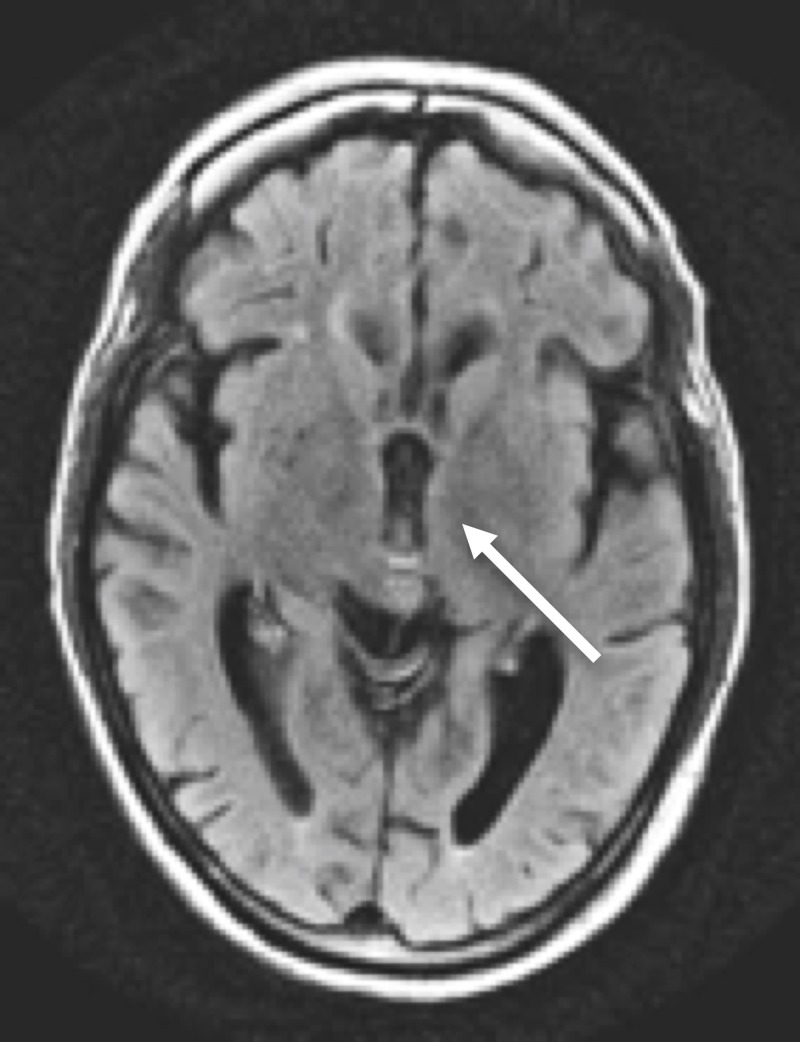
Axial FLAIR MRI of the head Axial FLAIR MRI of the head at the level of the 3^rd^ ventricle shows no significantly increased periventricular signal intensity (arrow)
